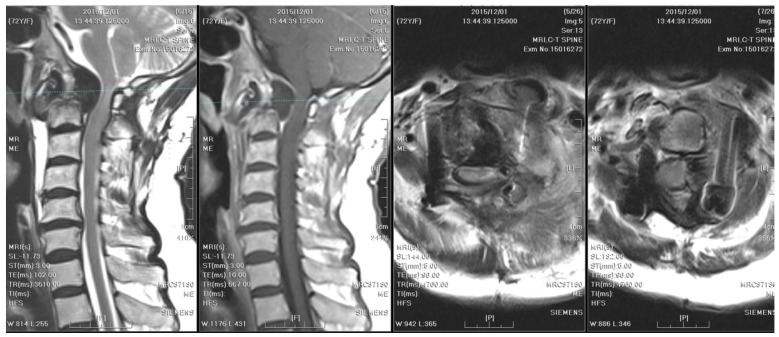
MRI of the cervical spine checked two years after the initial surgery demonstrates right rotatory atlantoaxial subluxation with bony destruction and surrounding soft tissue enhancement at the atlanto-odontoid joint, leading to severe spinal cord compression with focal myelomalacia. A thinner abscess wall (compared to the cervical spine MRI from two years ago), heterogeneously enhanced vertebral body, and subligamentous spread were observed.
